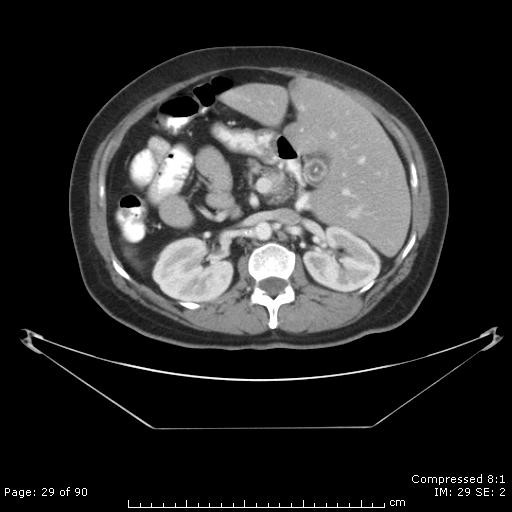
Pre-diagnosis CT scan. Consecutive CT scan slices demonstrate SI and mild atrophy of the pancreatic head but no mass present 8 months prior to her diagnosis of pancreatic cancer. The CT scan was obtained to evaluate abdominal pain.
